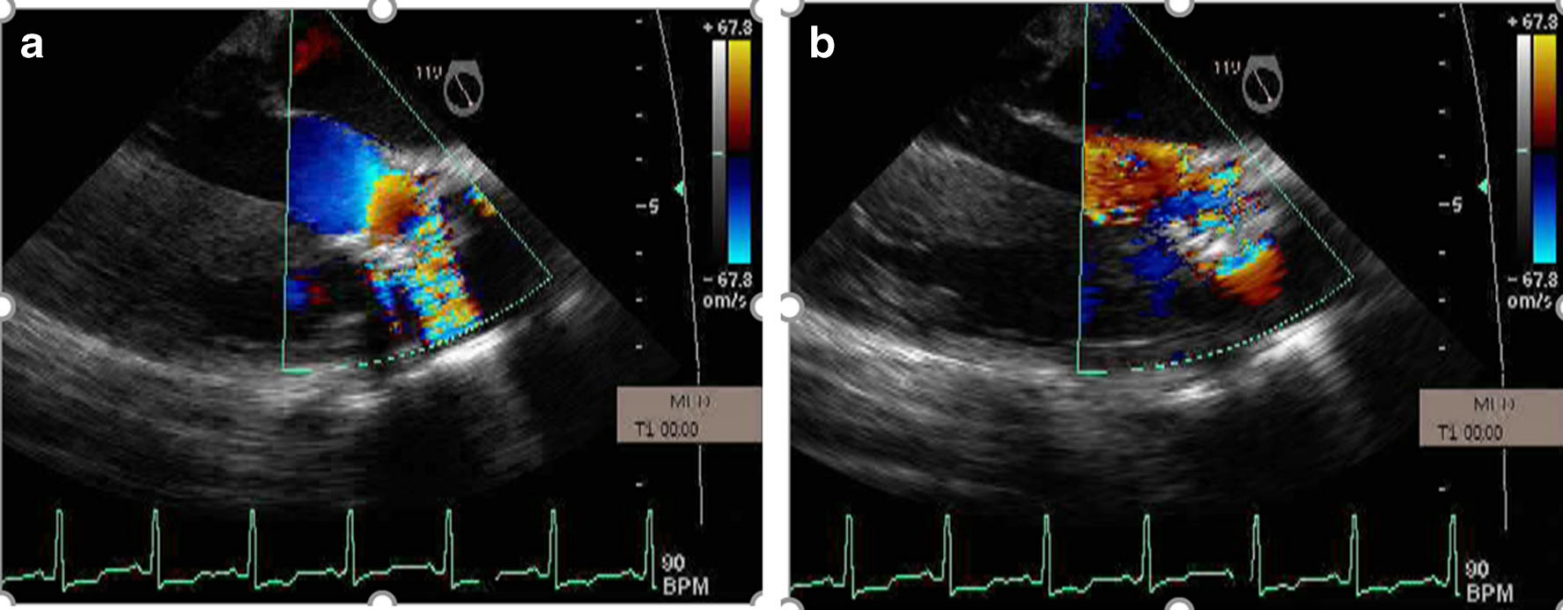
Transesophageal echocardiographic color Doppler images in the same patient as in Fig. 5 showing systolic Doppler flow convergence (PISA) toward the aortic valve at a normal aliasing velocity indicating a markedly increased gradient at the valve level from severe aortic stenosis in a and diastolic flow convergence (PISA) above the aortic valve at a normal aliasing velocity indicating severe aortic regurgitation in b. An emergent surgery was performed. Aortic leaflets were found fixed in mid open position. She underwent aortic valve replacement with a St. Jude Regent valve
